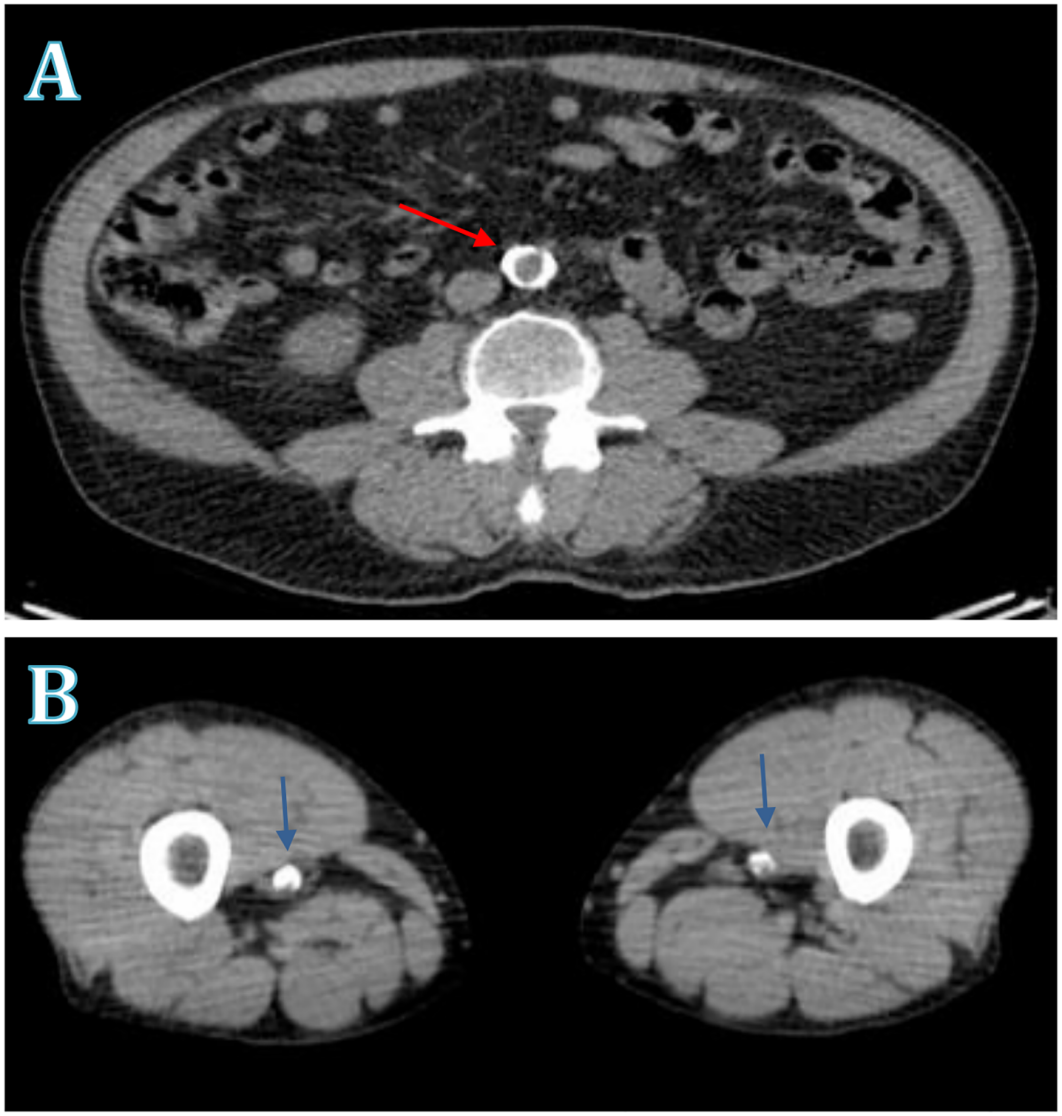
Arterial calcification. Unenhanced multi-sliced computed tomography images of arterial calcification (A) circumferential calcification of the distal abdominal aorta (B) calcification of both distal superficial femoral arteries at the level of the adductor hiatus with evidence of significant intraluminal stenotic plaque disease. Arrows identify areas of calcification.
